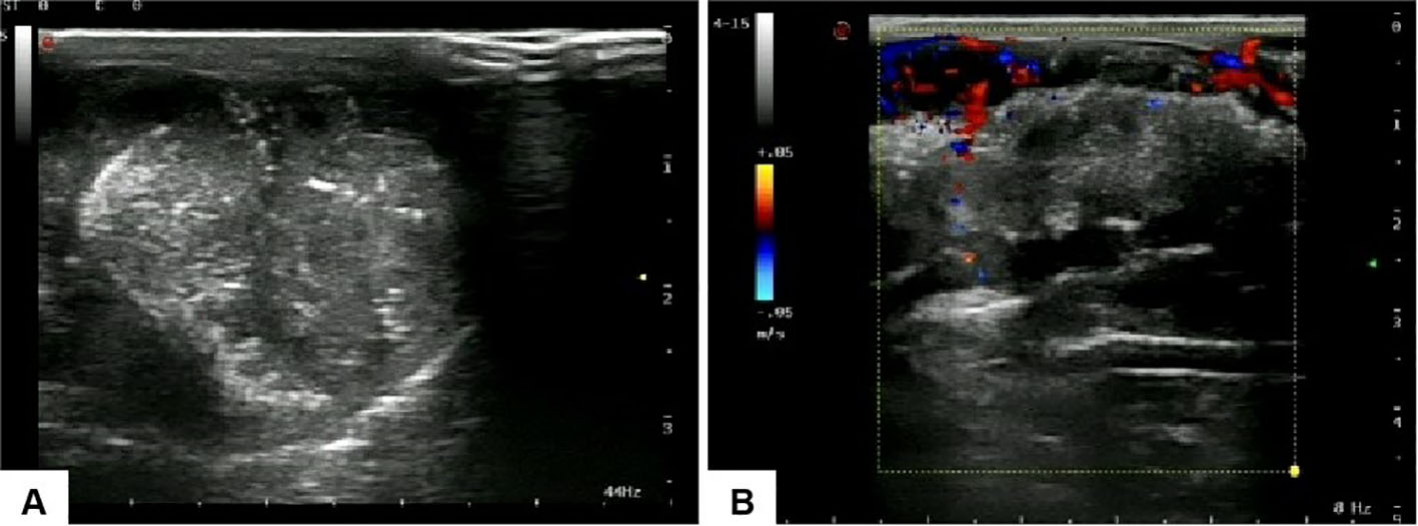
Ultrasound images of the penis: (A) clusters of calcified lesions are visible within the penile structure, and (B) fascicular blood flow signals are observed.
